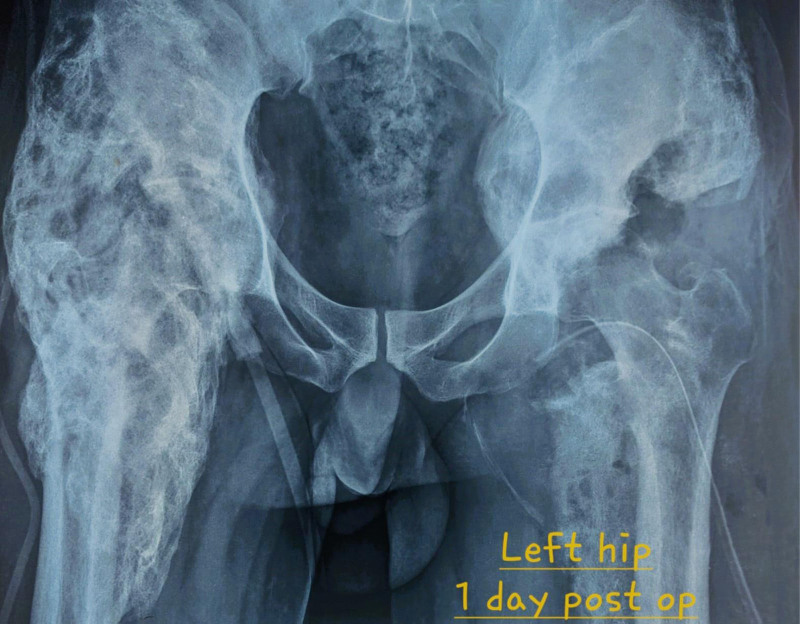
X-ray of the pelvis after surgery to remove the osteochondroma in the left hip (1 day post-op).
